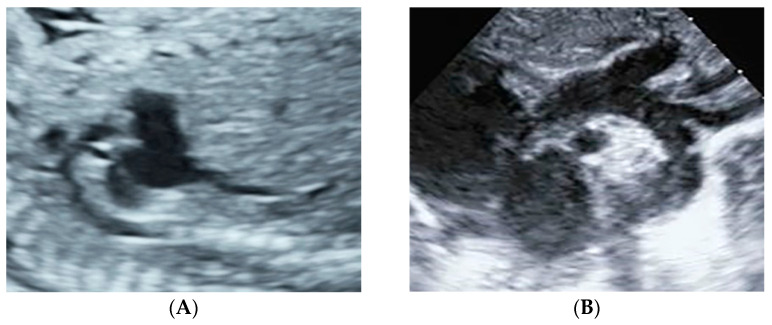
Prenatal and postnatal ultrasound images of the aortic arch. (A) At 32 + 6 weeks of gestation, the prenatal scan of the aortic arch showed a stiff and tortuous arch with a narrowed internal diameter, comparable to or slightly smaller than that of the adjacent carotid branches; the measurement yielded an aortic isthmus Z-score of 2.77 based on gestational age reference formulas (higher values indicate smaller isthmus diameters in our Z-score convention); (B) Postnatal imaging confirmed normal development of the aortic arch.
